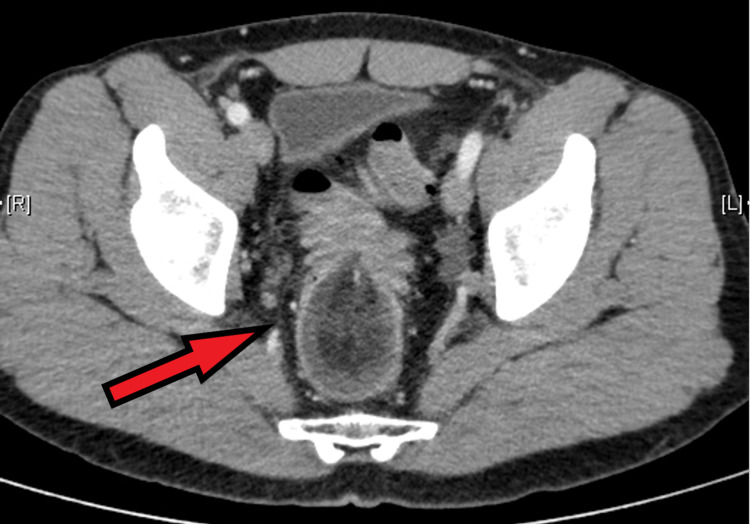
CT scan of the abdomen showing the colorectal intussusception
